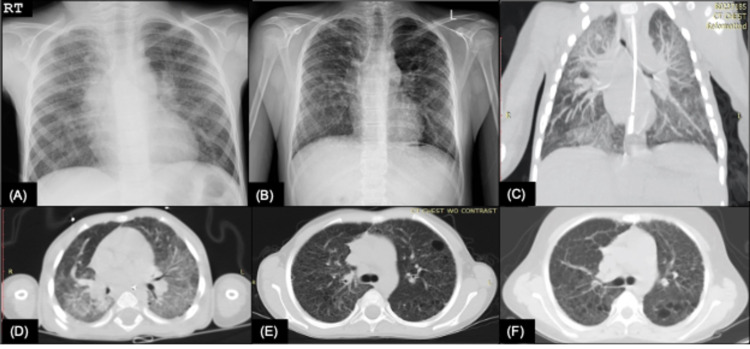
Progression of radiologic findings in a Puerto Rican male with SP-C deficiency. (A) AP view CXR taken at seven years of age showing central peribronchial wall thickening with bilateral diffuse interstitial changes. (B) PA view CXR taken at 13 years of age showing reticulonodular interstitial opacities. (C) Coronal and (D) transverse HRCT obtained at 14 months of age with evidence of significant ground-glass opacities, more prominent on the posterior lung aspects. (E) Transverse HRCT with decreased ground-glass opacity attenuation with a myriad of pulmonary cysts at six years of age and (F) 13 years of age. AP: anteroposterior; CXR: chest radiography; PA: posteroanterior; HRCT: high-resolution computed tomography
